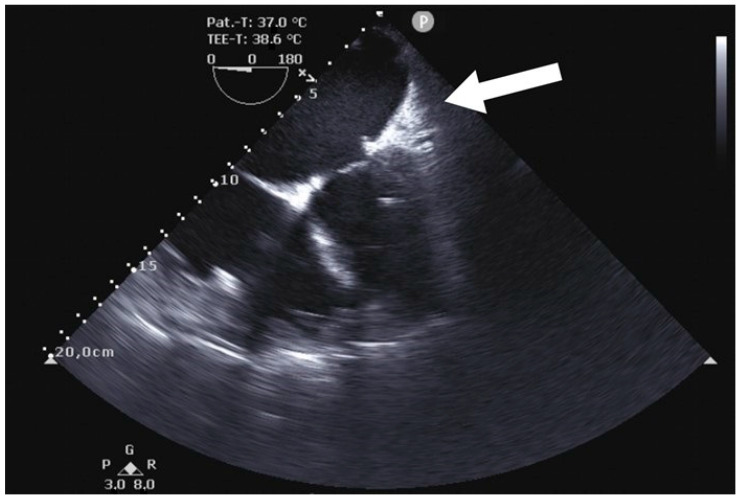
Postoperative transesophageal echocardiography showing the complete occlusion of LAA after correct placement of the AtriClip-Pro^®^ (AtriCure, Mason, OH, USA) device (P: Pointer on ultrasound transducer).
